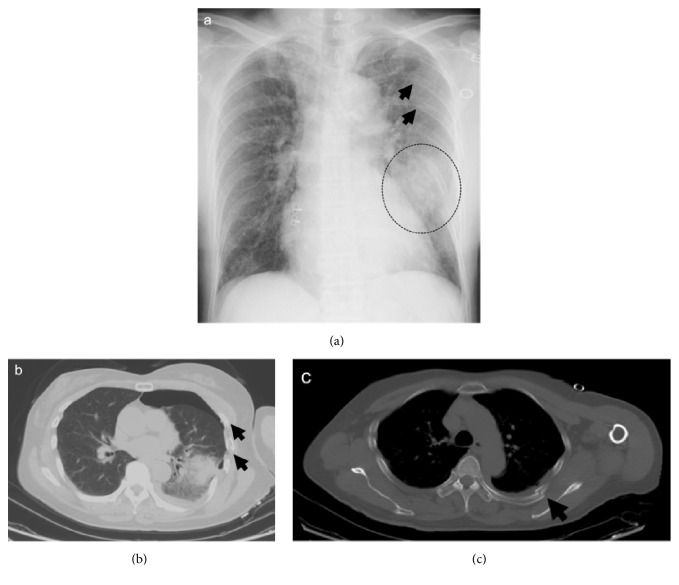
(a) Chest roentgenogram showing pulmonary contusion (dotted circle) with rib fractures (black arrows). (b and c) Chest CT showing left hemopneumothorax and pulmonary contusion with multiple rib fractures (black arrows).
